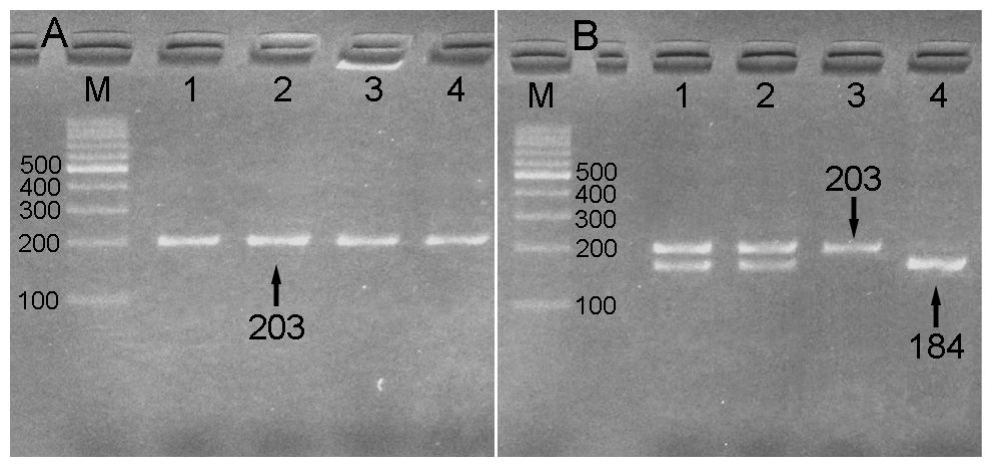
Electrophoresis of PCR products of the samples and genotyping of the *IL*-8 +781C/T SNP. (A) *IL*-8 +781C/T SNP: lane M, 100 bp marker ladder, lanes 1–4, samples, the 203 bp bands are the PCR products. (B) genotyping of the *IL*-8 +781C/T SNP: lane M, 100 bp marker ladder; lanes 1 and 2, CT genotype (203-, 189- and 19-bp); lanes 3, TT genotype (203 bp); and lanes 4, CC genotype (184- and 19-bp). The 19 bp fragment was invisible in the gel owing to its fast migration speed.
